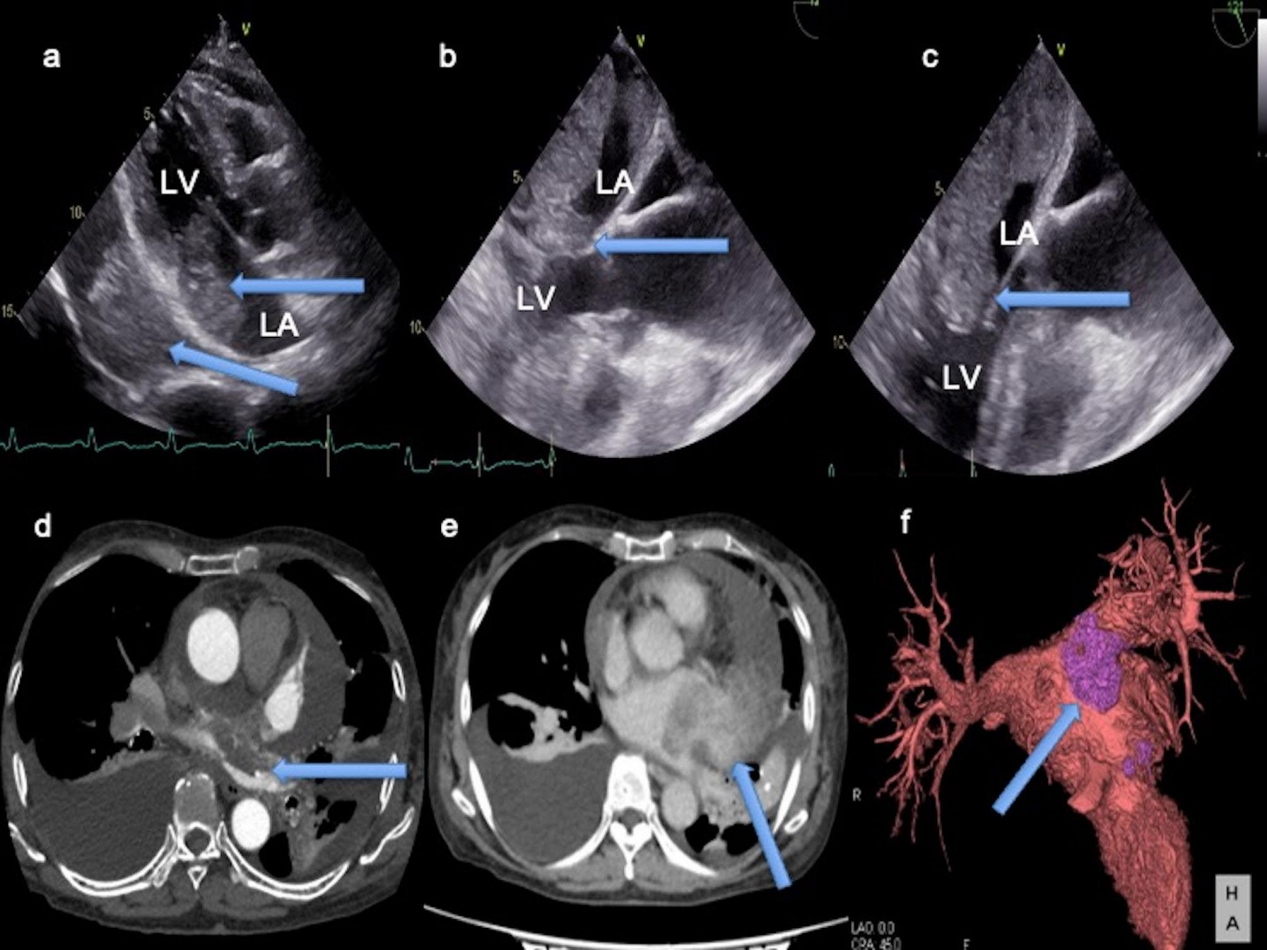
Preoperative transthoracic echocardiogram (TTE), transesophageal echocardiogram (TEE), and computed tomography (CT) images (a) TTE revealing a movable mass in the left atrium (LA) and another mass in the pericardium. (b) TEE showing the tumor in the LA during the systolic phase. (c) LA tumor prolapsed across the mitral valve during the diastolic phase. (d, f) CT and three-dimensional CT revealing a mass in the LA extending to the left upper PV, (e) Both masses were heterogeneously different. LV: left ventricle
